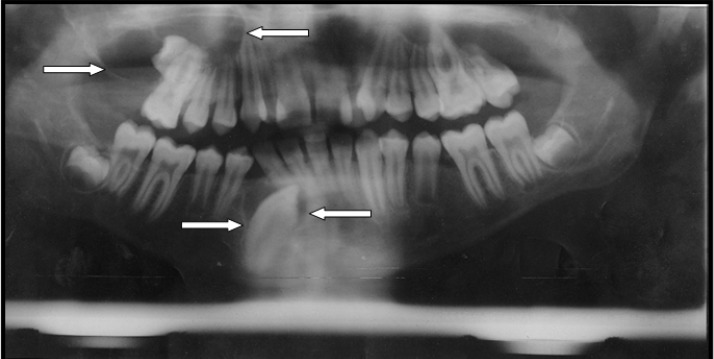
Panoramic radiograph showing two cyst-like radiolucencies in upper and lower jaws
